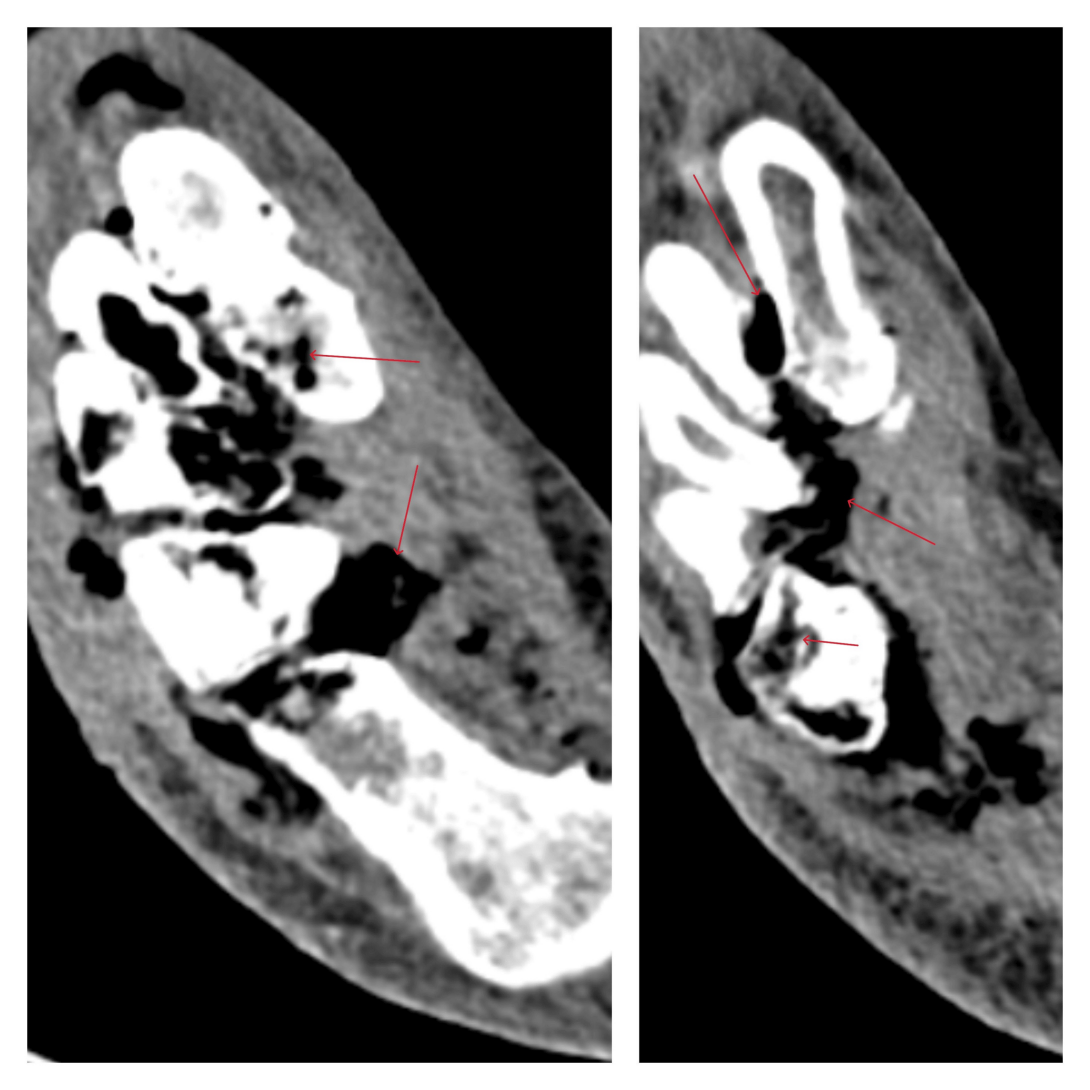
Axial contrast-enhanced computed tomography images of the right foot demonstrating extensive soft tissue gas tracking along the fascial planes (arrows), with associated soft tissue edema and osseous involvement, consistent with necrotizing soft tissue infection and underlying osteomyelitis.
